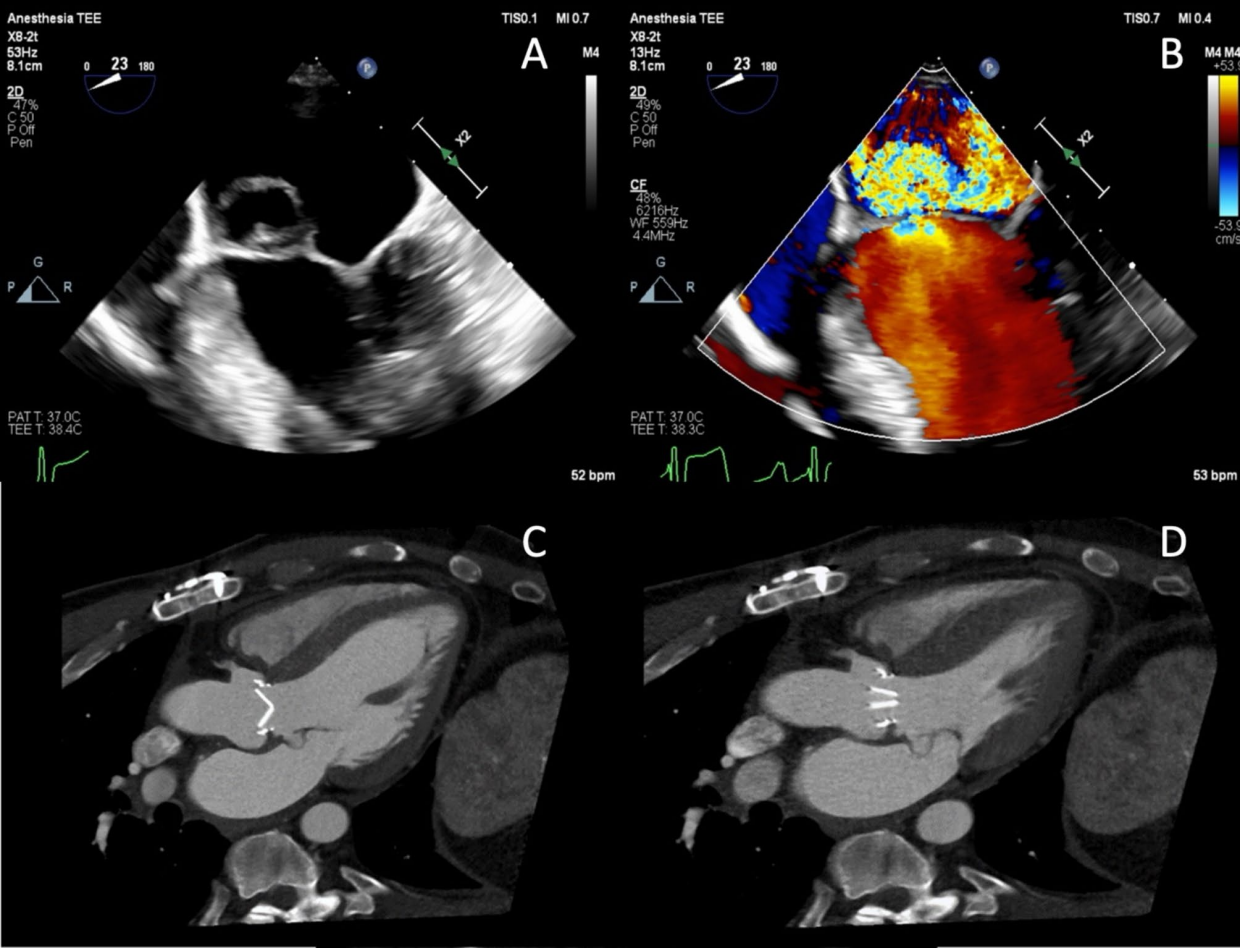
A windsock deformity of the anterior mitral valve leaflet is shown using (A-B) transesophageal echocardiography, as well as computed tomography angiography in (C) diastole and (D) systole
